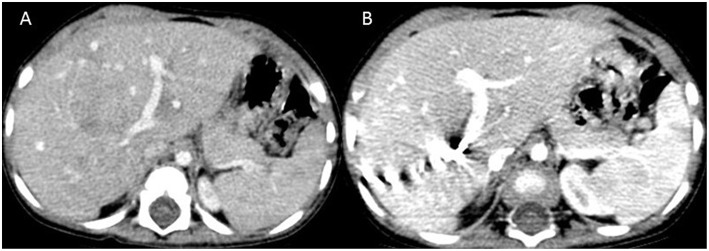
Computed tomography images at the level of splenic hilum before (A) and after (B) portal vein embolization showing hypertrophy of segment 3.
